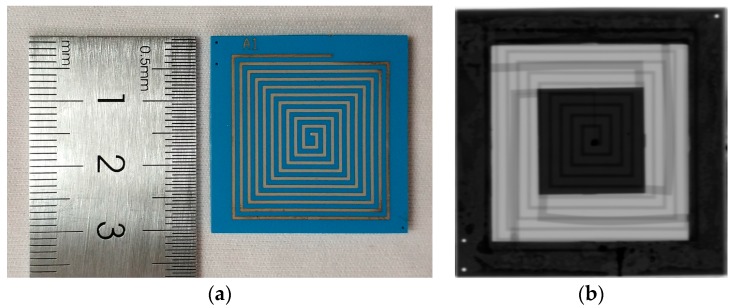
Fabricated LTCC accelerometers: (a) optical image; and (b) X-ray inspection image.
